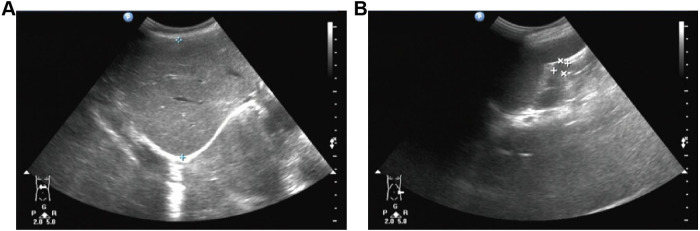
Abdominal ultrasound. (A) Splenomegaly; (B) Hepatomegaly.
